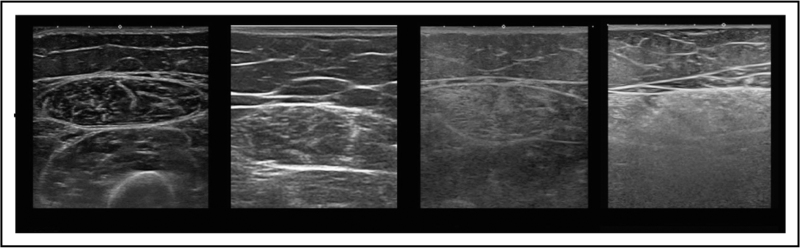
Visual (qualitative) evaluation of muscle ultrasound. Muscle ultrasound of quadriceps (rectus femoris and vastus intermedius) muscle, showing different degrees of muscle abnormalities from left (normal MUS with *starry night* appearance) to right (severe abnormalities). The four images correspond to grades 1 to 4 on the Heckmatt scale, respectively.
